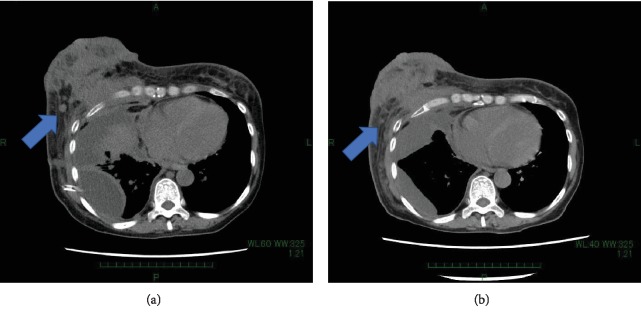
(a) Breast sections of CT scan before initiation of dabrafenib and trametinib. (b) Same section 2 weeks after treatment initiation. The arrow points to reduced mass and decreased pleural effusion.
